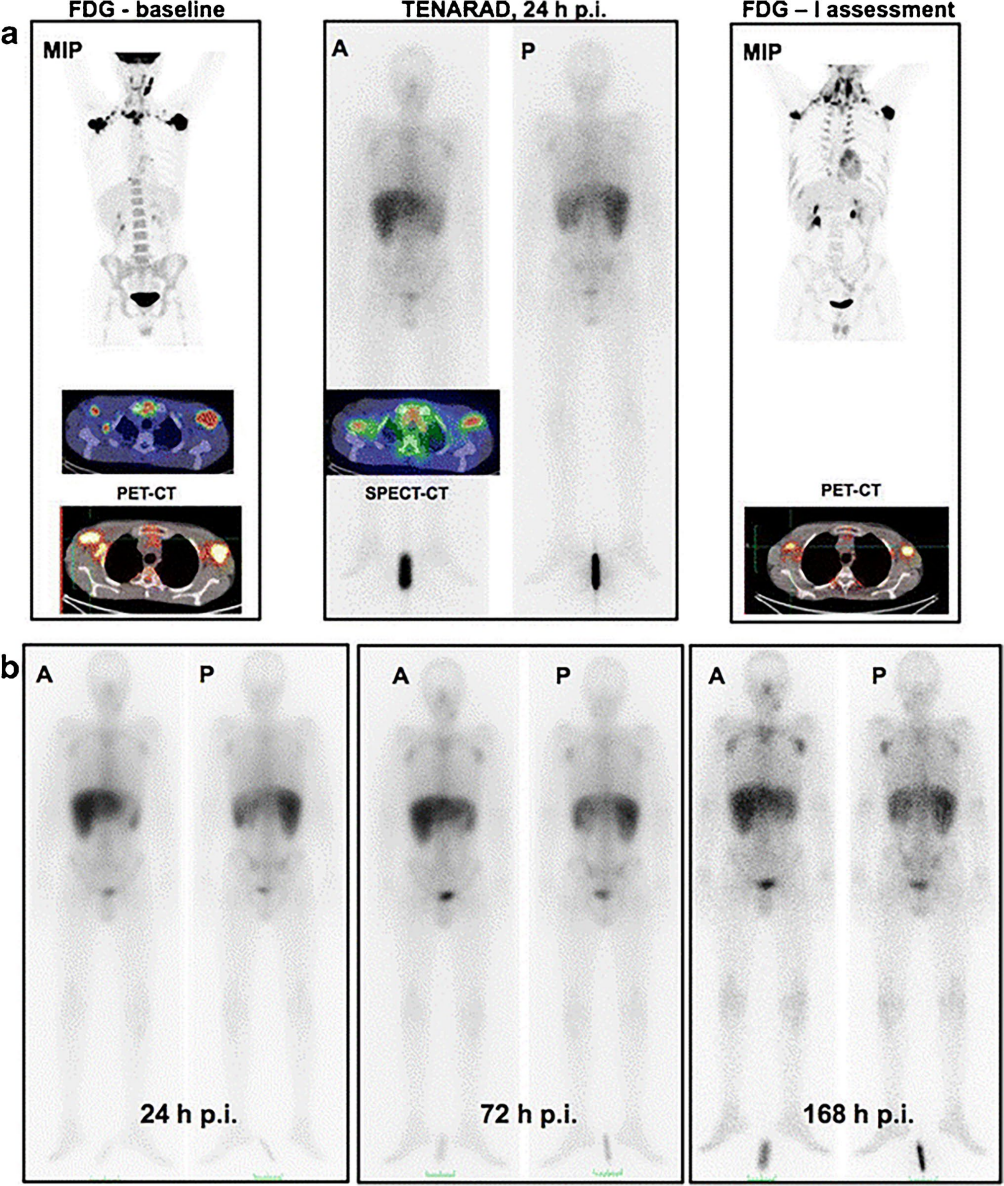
PET/CT scans of 1/5 responding Hodgkin’s lymphoma patients treated with the TNC-A1-targeting radioligand [^131^I]I-F16SIP (Tenarad). a Maximum intensity projection image of the baseline FDG PET/CT scan (left), the [^131^I]I-F16SIP SPECT/CT scan 24 h post-injection (middle), and the FDG PET/CT scan 4 weeks after [^131^I]I-F16SIP treatment (right). b Whole-body images at different time points after 3.33 GBq [^131^I]I-F16SIP administration, demonstrating uptake in tumor lesions. *A: anterior view*, *P: posterior view.* The figure was originally published in EJNNMI by Aloj et al. [65]. Radioimmunotherapy with Tenarad, a 131I-labeled antibody fragment targeting the extradomain A1 of tenascin-C, in patients with refractory Hodgkin's lymphoma (2014) Vol 41(5):867–877
